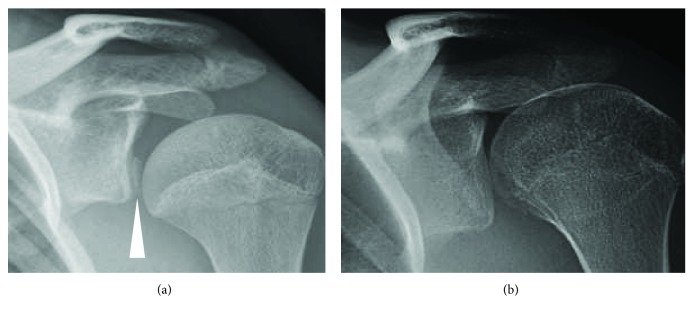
Anteroposterior plain film radiographs of the patient's left shoulder. Bony fragment off the anterior glenoid preoperative indicated by arrow (a). Successful anatomical reduction of the bony fragment four months postoperative (b).
